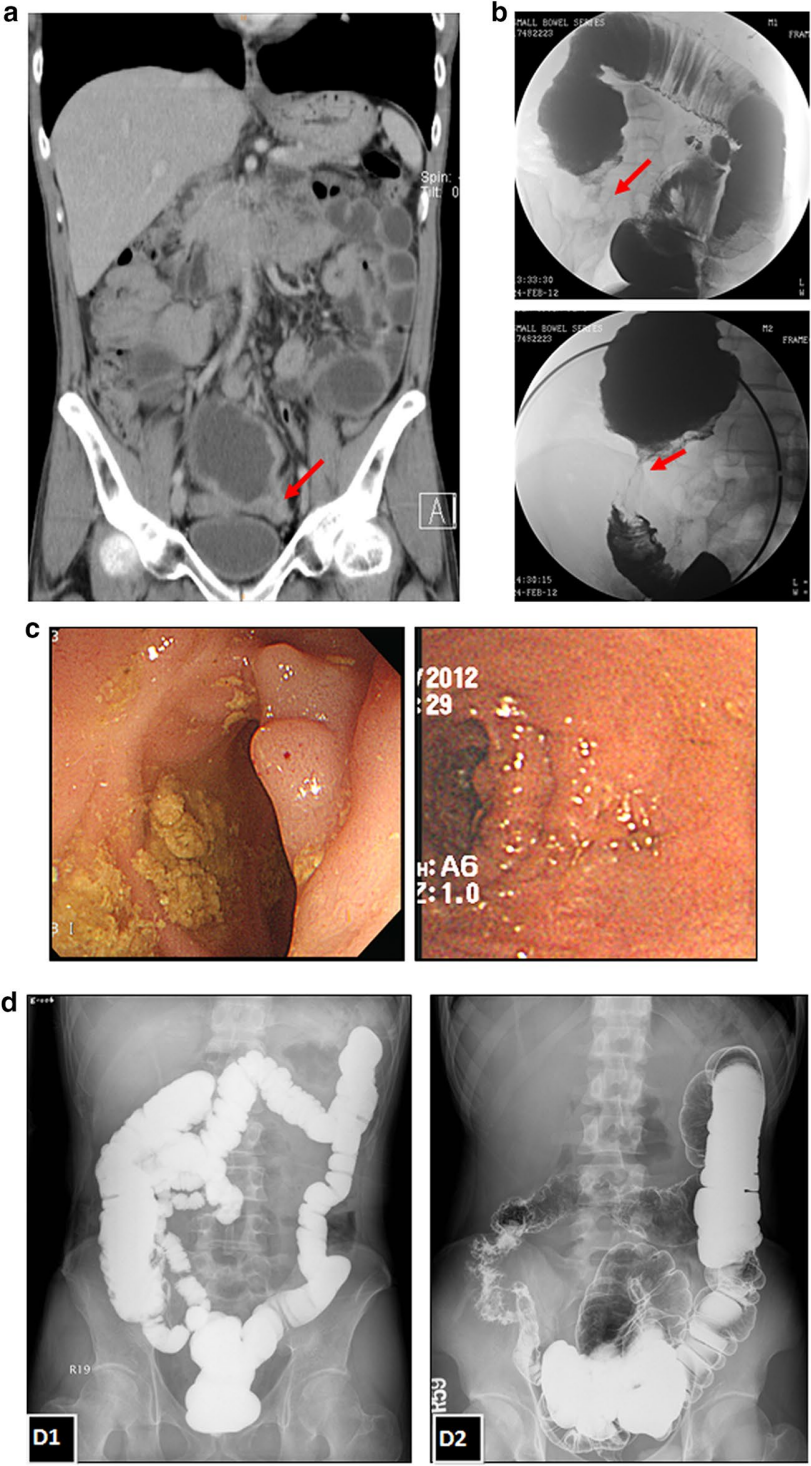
Image studies of the patient with intestinal tuberculosis initially misdiagnosed as Crohn’s disease. a–c Images for making the initial diagnosis of Crohn’s disease. a Coronal view of abdominal computed tomography showed skip lesions with interrupted dilatation of the small bowel, and one stricture point at the terminal ileum with the dilated proximal intestine (*arrow*); b Initial barium radiographic study of the intestine showed segmental narrowing of the ileum, which favored post-inflammatory focal stricture (*arrow*); c Colonoscopic examination showed polypoid lesion at the terminal ileum. d
